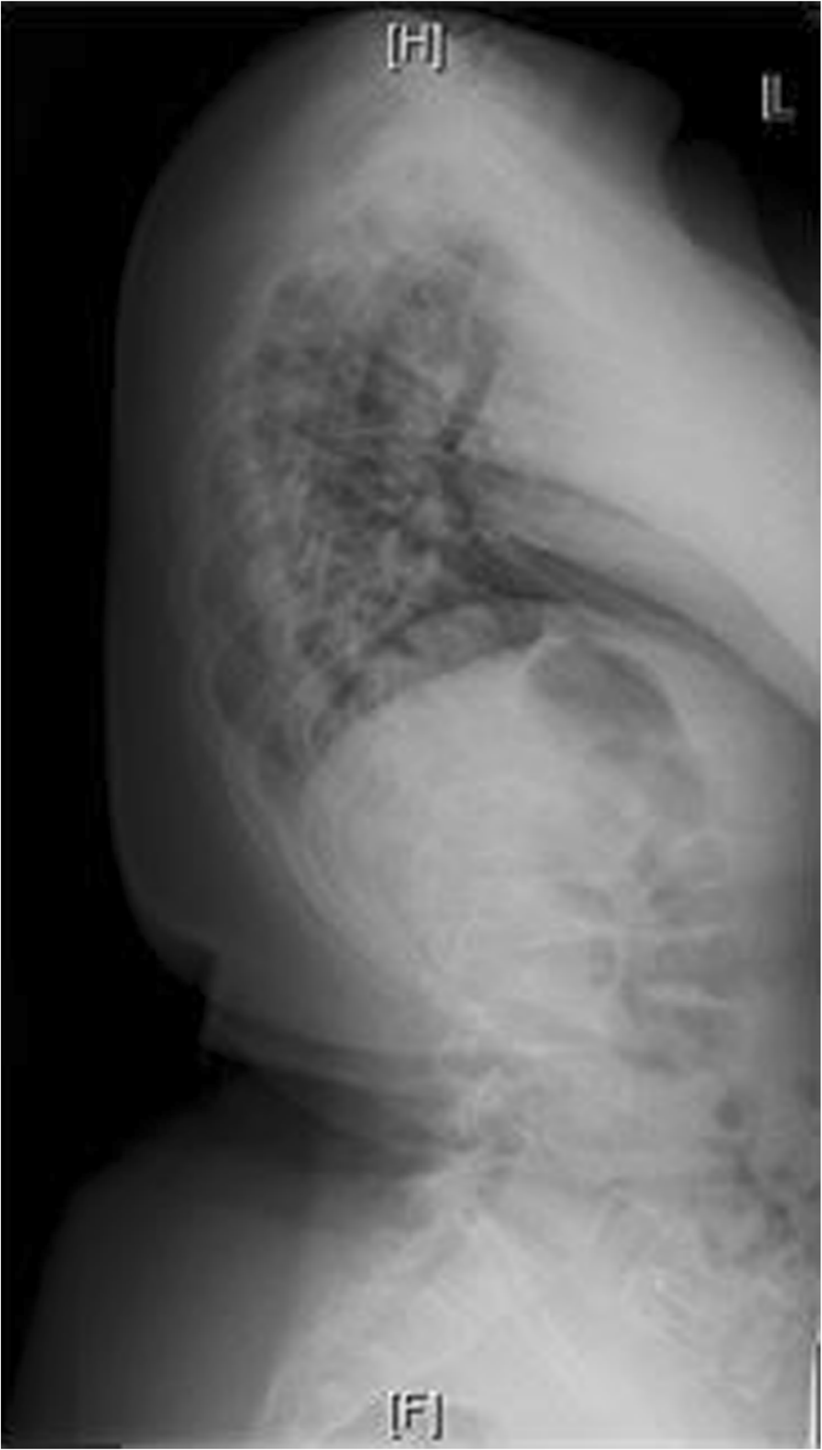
Preoperative radiograph of a 14 year old with Prader-Willi syndrome.
